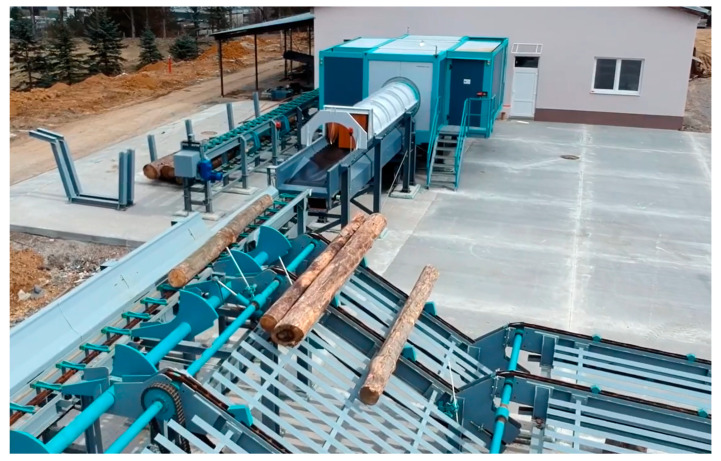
CT LOG X-ray Computer Tomography scanner—National Forest Center Zvolen—Biotechnological Park Stráž.
